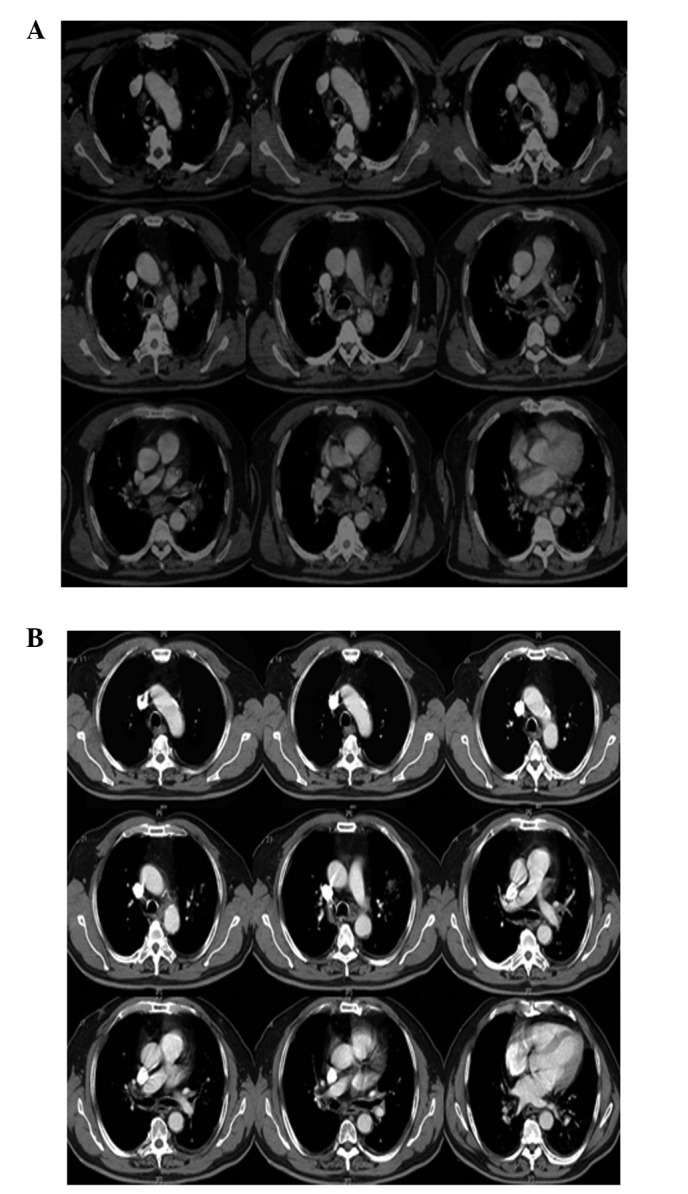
Multiple mediastinal tumors (15 lesions). CT revealed that the tumors disappeared following CyberKnife treatment. (A) CT prior to CyberKnife therapy (January 28, 2011); (B) CT following CyberKnife therapy (March 21, 2011).
